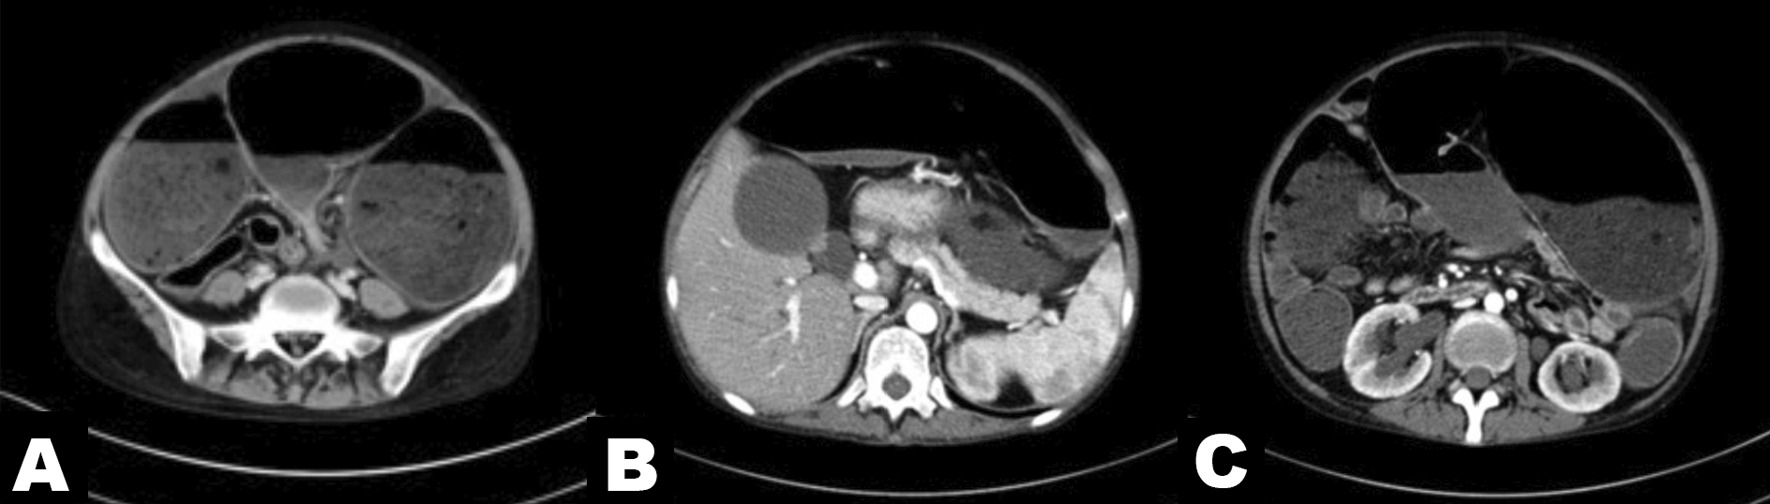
Computed tomography scans of the abdomen. Localized stenosis of the sigmoid colon and general dilation of the bowel (A); dilated intra- and extra-hepatic bile ducts, dilated pancreatic ducts and enlargement of the gallbladder (B); dilation of the renal pelvis, calyces and ureter (C)
